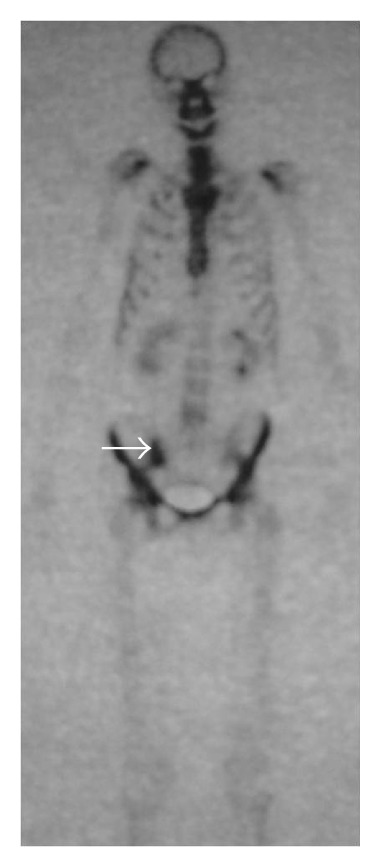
Technetium-99m MDP bone scintigraphy of the anterior pelvis shows increased uptake in the right sacroiliac joint (arrow).
